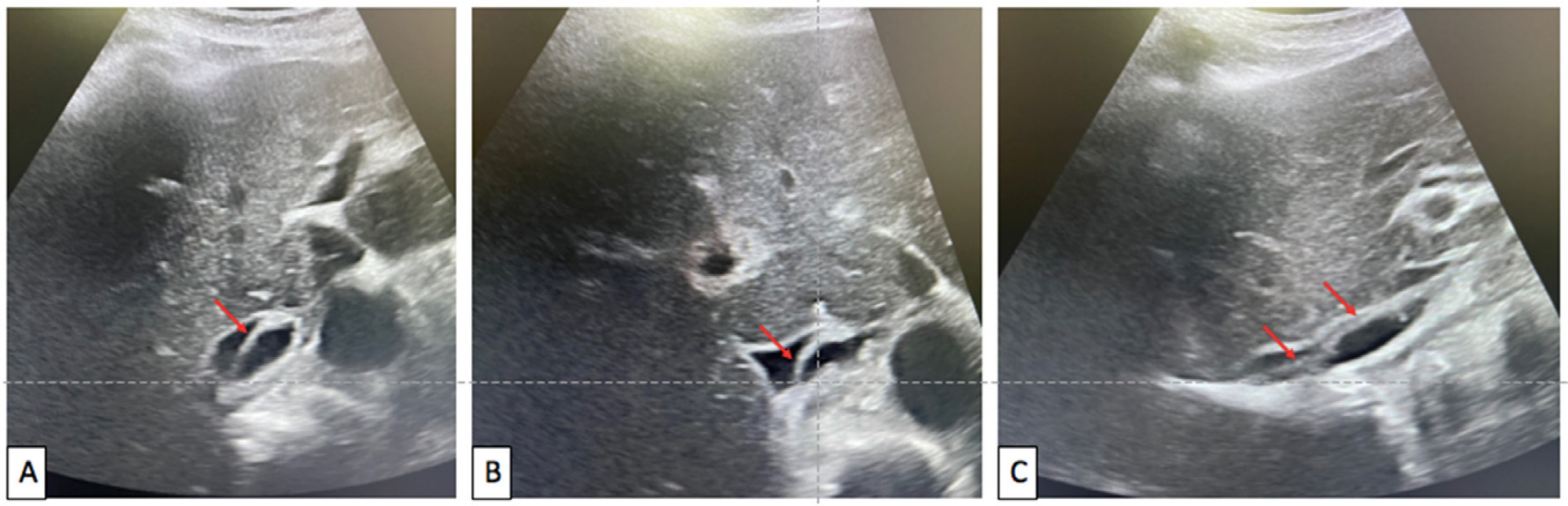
Vena cava ultrasound in B-mode in axial (A,B) and sagittal (C) view: Red arrows point the endoluminal web inside the retro-hepatic vena cava.
